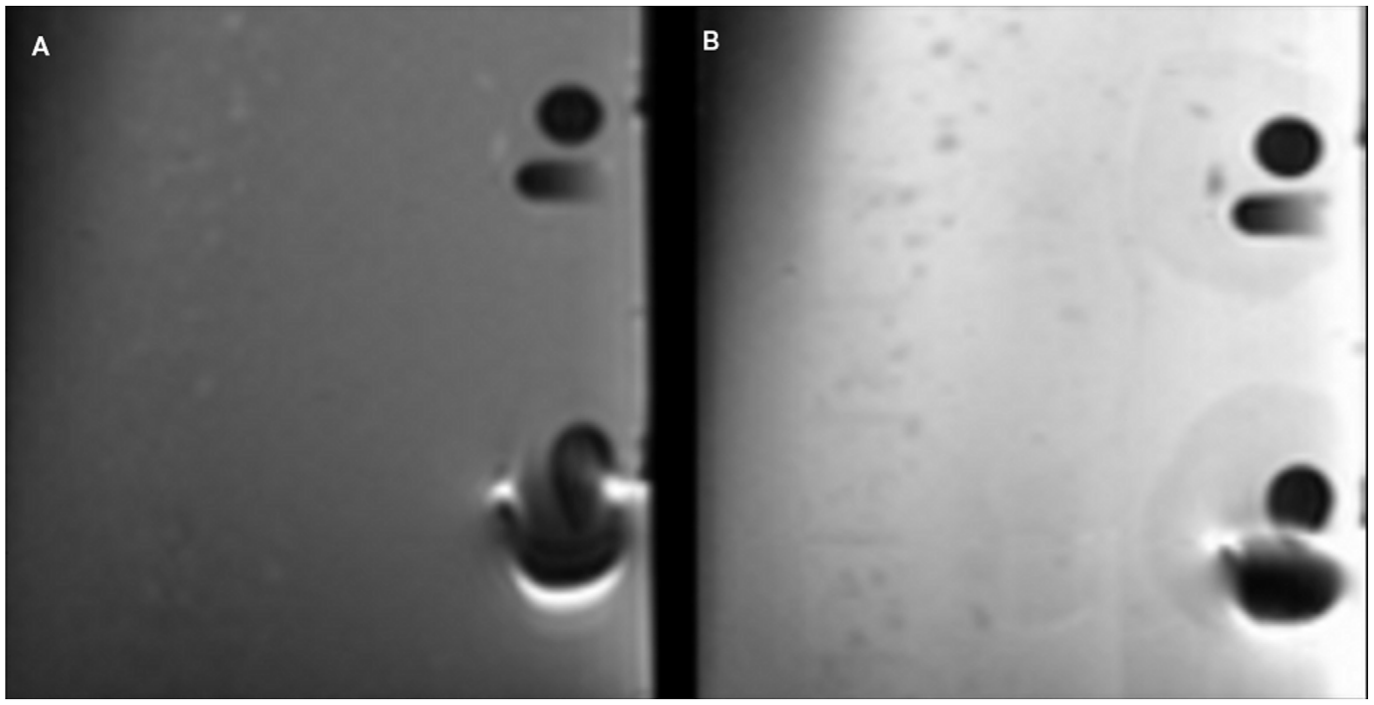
MRI artifact produced by Tetric EvoCeram in (A) T1 and (B) T2.
